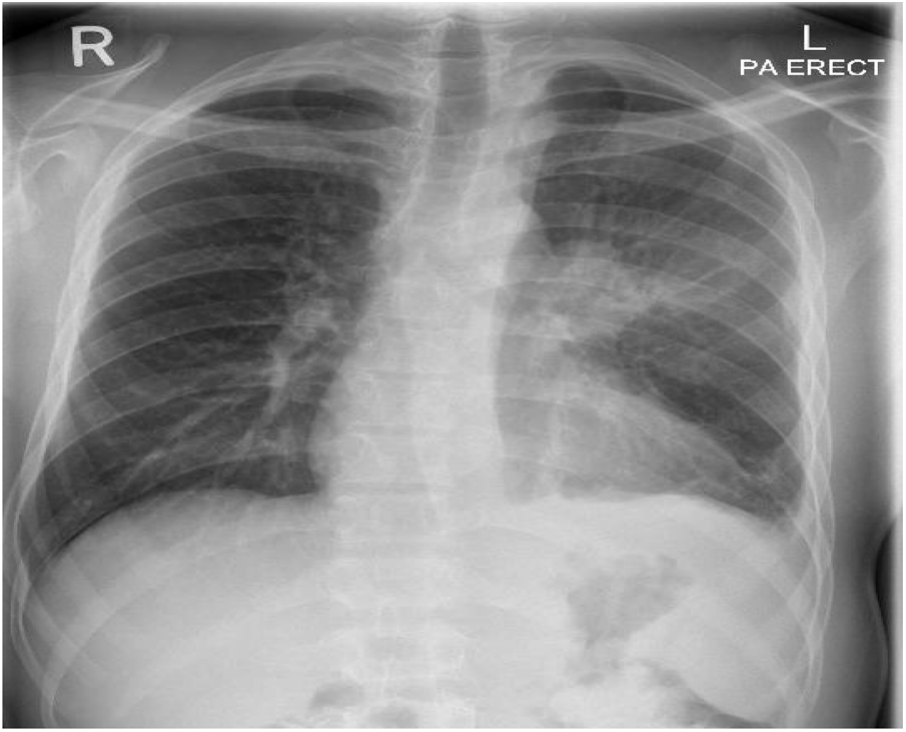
Chest x-ray showed airspace opacity is noted in the left perihilar region.
